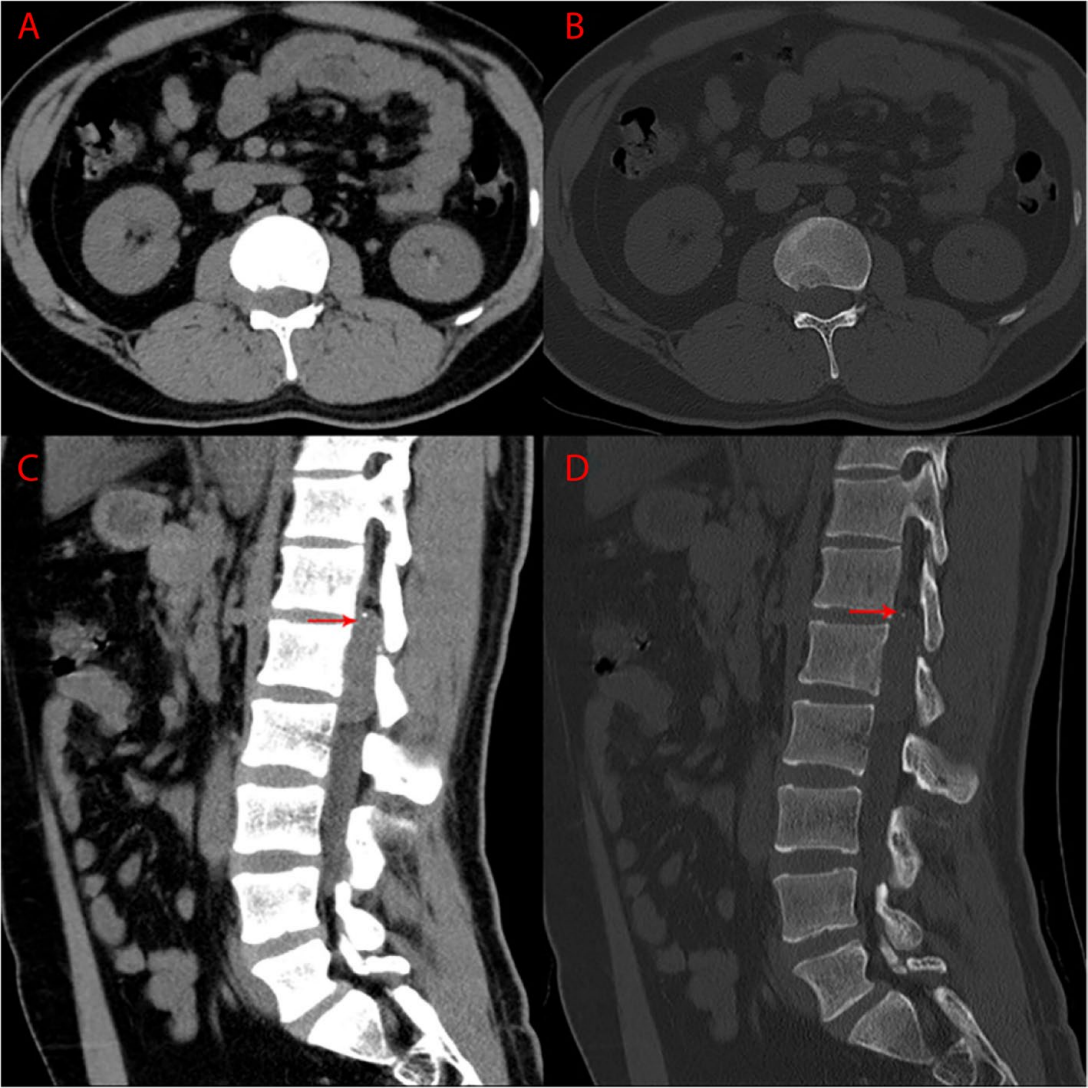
Preoperative CT images. A, B Axial images. C, D Sagittal images. CT images revealed isolated punctate calcification in the mass (arrows)
